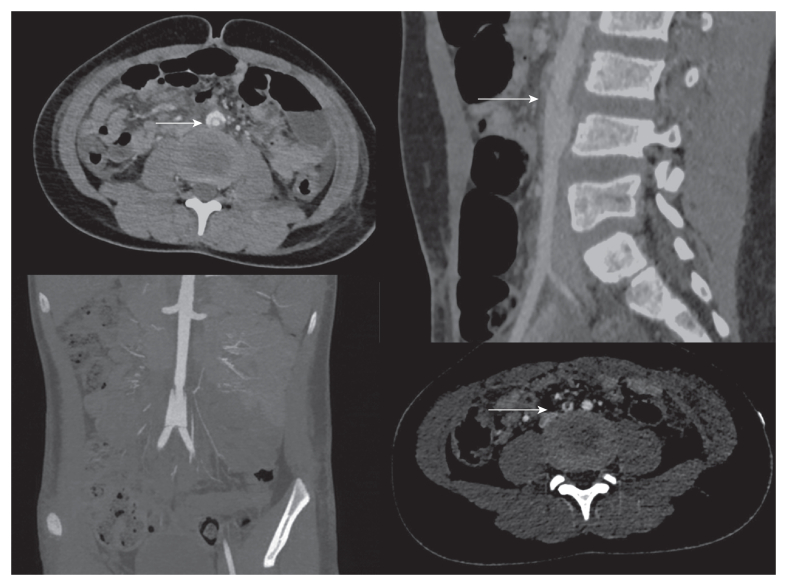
Abdominal computed tomography angiography performed 48 hours after the trauma. Top left: Axial slice showing circumferential intimal dissection of the infrarenal abdominal aorta. Top right: Sagittal slice showing floating intimal flap (arrow) with ectasia measuring 14 mm. Bottom left: Coronal slice showing intimal dissection. Bottom right: Axial slice showing intimal dissection at common iliac origins.
